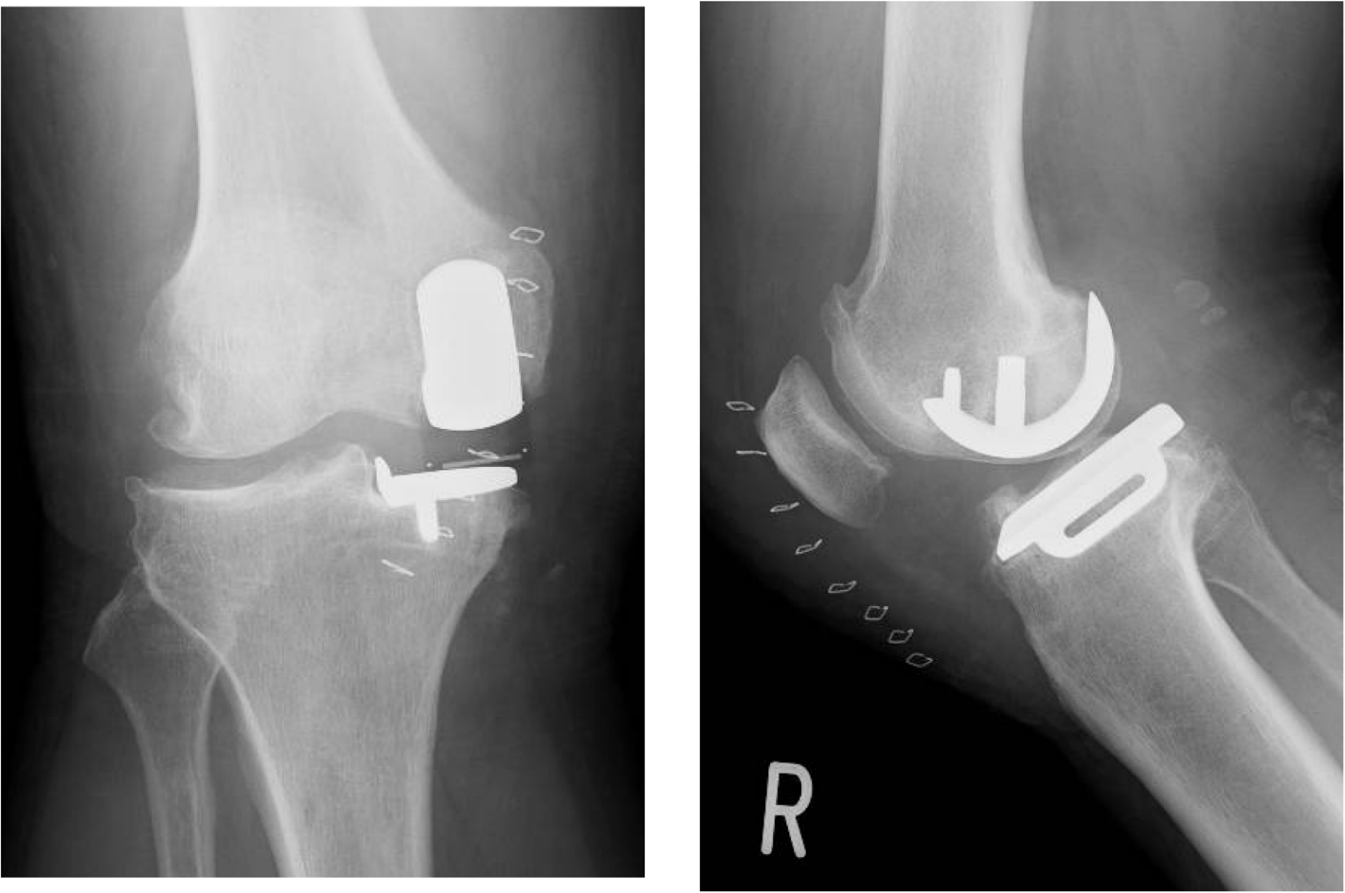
Radiographs of case 2 after the additional surgery showing femoral migration and radiolucent zone around the femoral pegs
